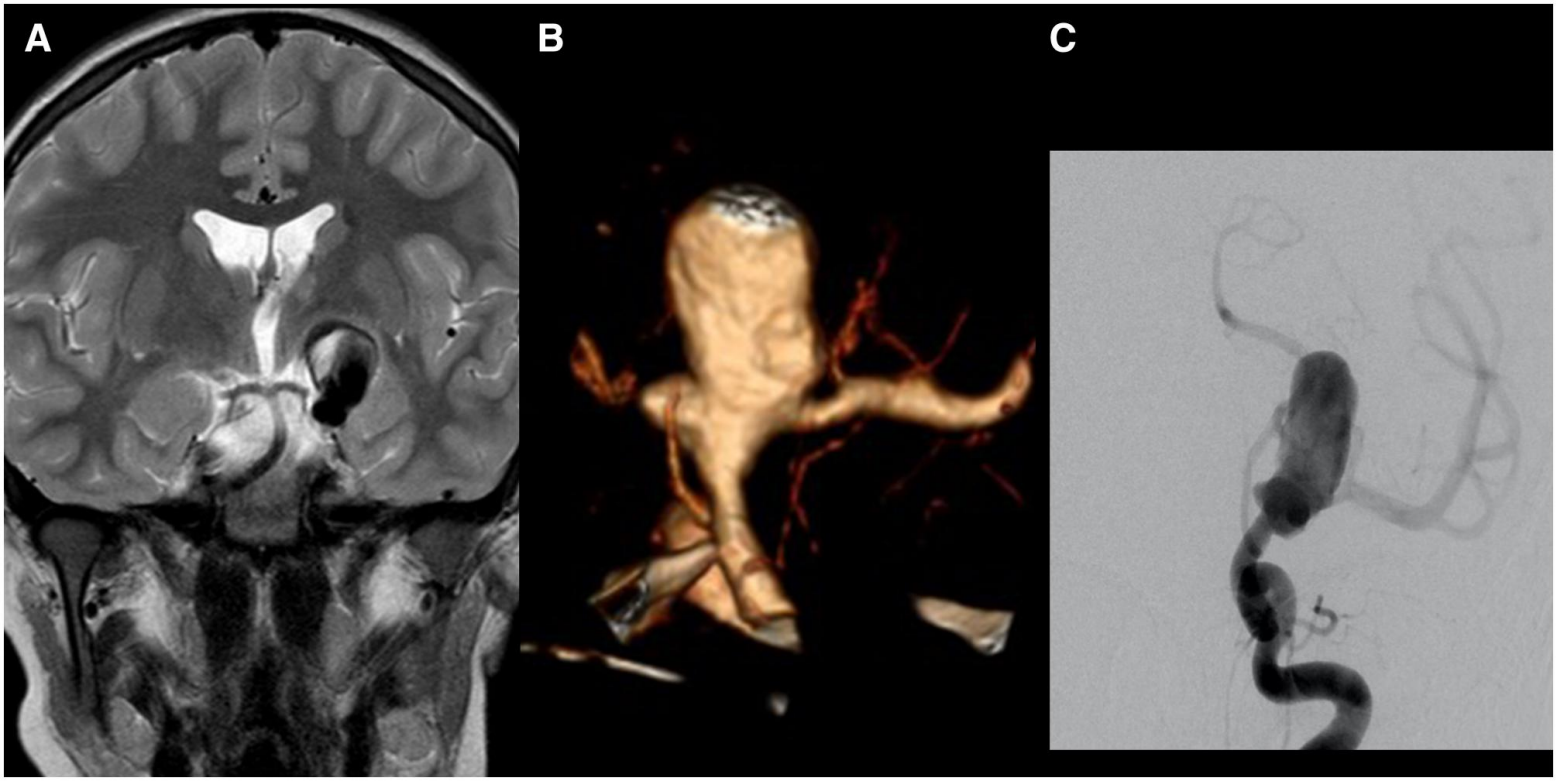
(A) Coronal T2-weighted image of an MRI performed for unspecific headache in the then 10-year-old boy. It shows a giant and partially thrombosed aneurysm of the left ICA. There is no oedema but mass effect on the third ventricle. (B) The CTA shows the broad-based aneurysm incorporating the carotid termination. There is no A1 segment. The left M1 segment originates from the aneurysm. A smaller lobule is seen pointing medially. The internal surface of the aneurysm appears coarse. (C) The diagnostic angiogram shows contrast pooling in the aneurysm before filling theMCA. Abbreviation: ICA = internal carotid artery; CTA = CT angiography; MCA = middle cerebral artery.
